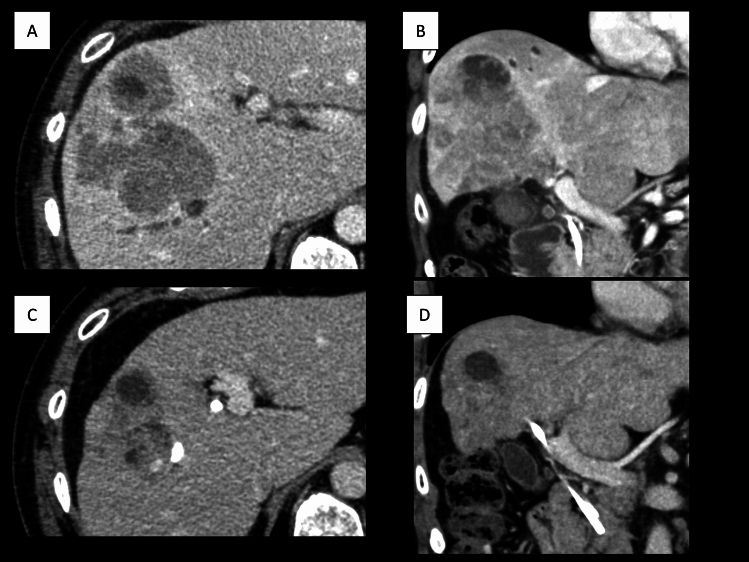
Abdominal computed tomography before pemigatinib treatment (A, B) and after 6 months of pemigatinib treatment (C, D); A and B show an increase in tumor size despite 19 months of conventional chemotherapy; C and D show tumor size reduction and the disappearance of tumor thrombi in the common bile duct after 6 months of pemigatinib treatment
